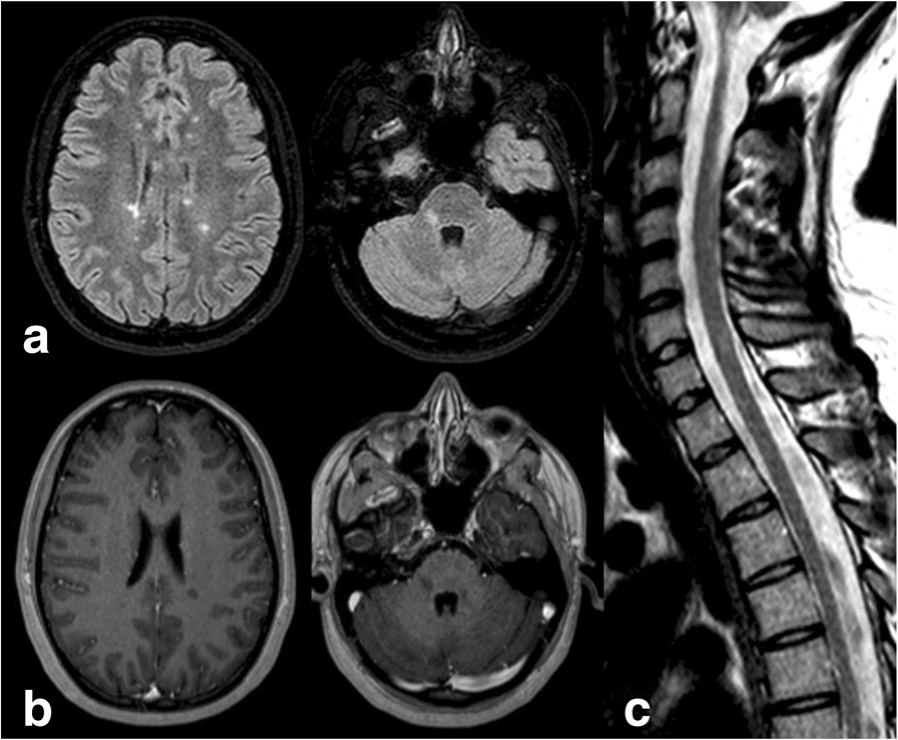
Axial (a) T2-weighted FLAIR MRI under stable clinical conditions, showing several supratentorial lesions; axial (b) T1-weighted images showing no lesions with ring gadolinium-enhancement, (c) sagittal T2-weighted FLAIR MRI showing two spinal cord lesions
